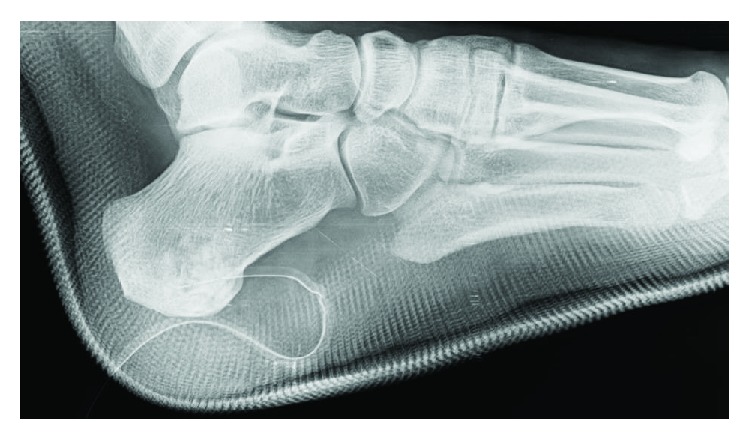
Postoperative lateral plain radiograph of the patient's foot shows radiopacity of the bone grafts at the surgery site.
